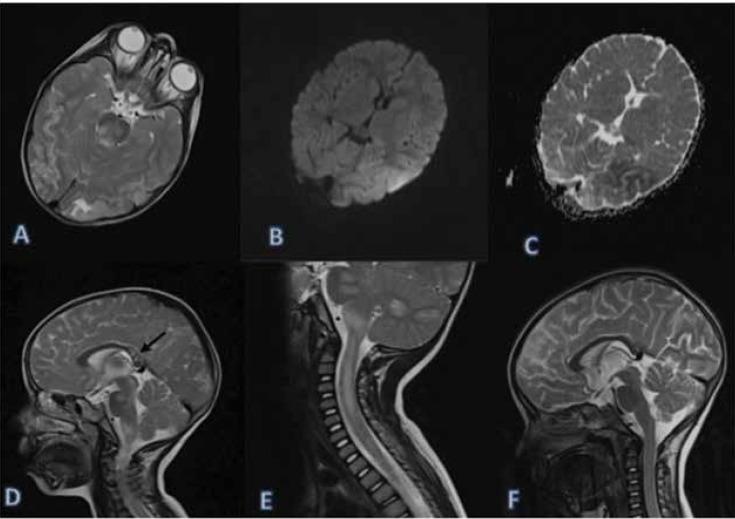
Axial brain MRI shows high signal intensities in the parietal and occipital regions, as well as the brainstem on T2-weighted images (A). Restricted diffusion on DWI and ADC maps (B, C). Sagittal T2-weighted images show abnormal signal intensities in the splenium of the corpus callosum (black arrow) and the brainstem (D). Abnormal signal intensities in the brainstem, cerebellum, and cervical cord (E). Normal follow-up MRI of the brain and spinal cord after one month (F)
